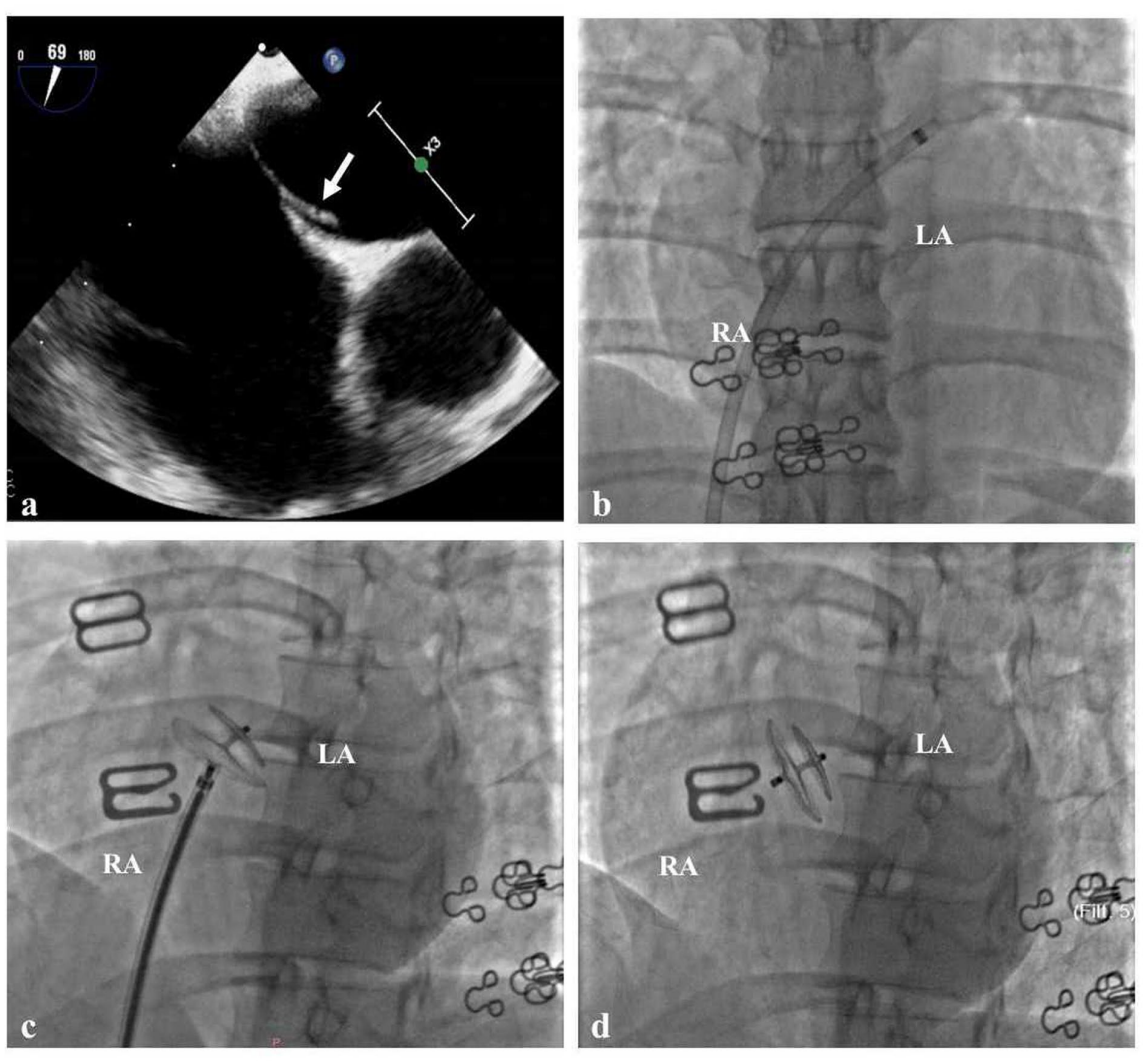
Cardiac Imaging in the Patient (A) Transoesophageal echocardiography showed that the patent foramen ovale was long tunnel-like (white arrow). (B) The delivery sheath passes through the PFO from the right to the left atrium. (C) The traction test of PFO closure was successful. (D) Made occluder successfully released.
